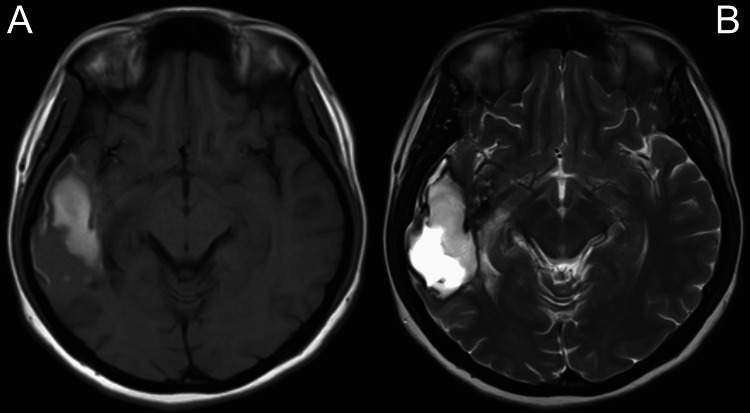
MRI of the brain, T1 sequence (A), and T2 sequence (B) showing right temporal lobe intra-axial cortical base lesions with adjacent mass effect and perilesional oedema.
